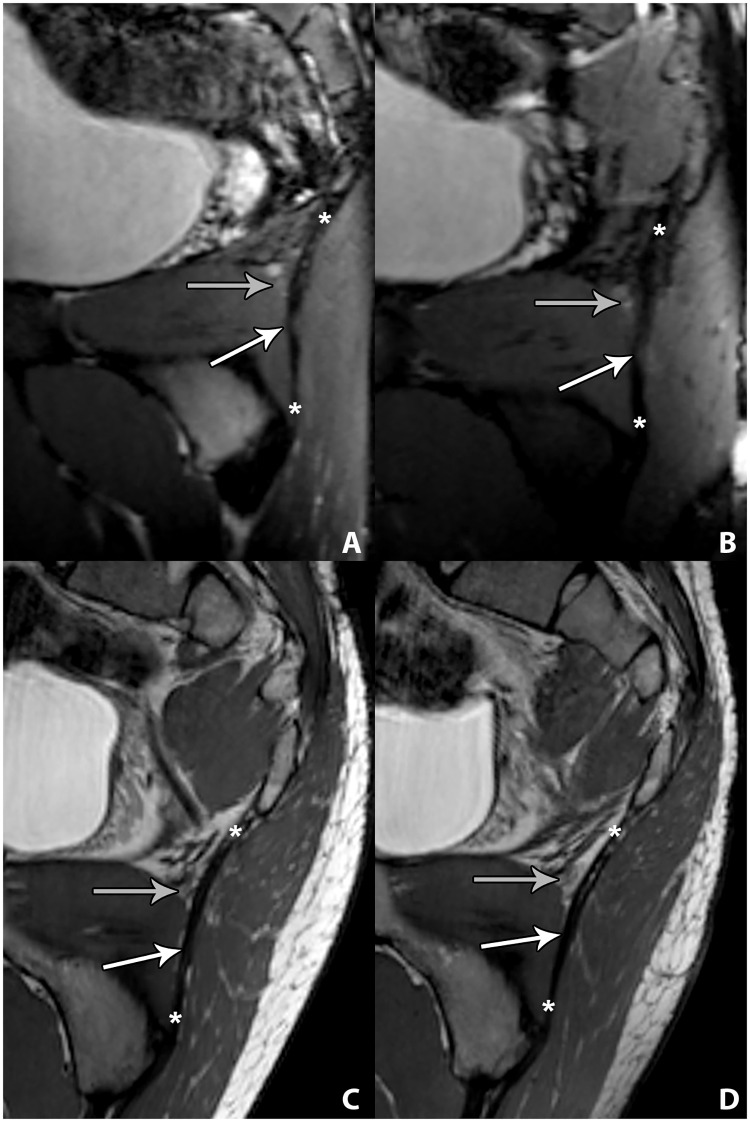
Post-operative pudendal nerve three-dimensional 3-Tesla MR Neurography of the sacrotuberous ligament 3 months (A) and 8 months (B) following sacrotuberous ligament division. A: Sagittal oblique multiplanar T2-weighted MR images with fat suppression demonstrate the continuous left sacrotuberous ligament (STL) (asterisks) with healing division site (white arrow). The gray arrow demonstrates the pudendal nerve subjacent to the STL. B: Sagittal oblique multiplanar T2-weighted MR images with fat suppression demonstrate the continuous right sacrotuberous ligament (STL) (asterisks) with healing division site (white arrow). The gray arrow demonstrates the pudendal nerve subjacent to the STL. C: Sagittal oblique multiplanar intermediate-weighted MR images demonstrate the continuous left STL (asterisks) with healed division site (white arrow). The gray arrow demonstrates the pudendal nerve subjacent to the STL. D: Sagittal oblique multiplanar intermediate-weighted MR images demonstrate the continuous left STL (asterisks) with healed division site (white arrow). The gray arrow demonstrates the pudendal nerve subjacent to the STL.
